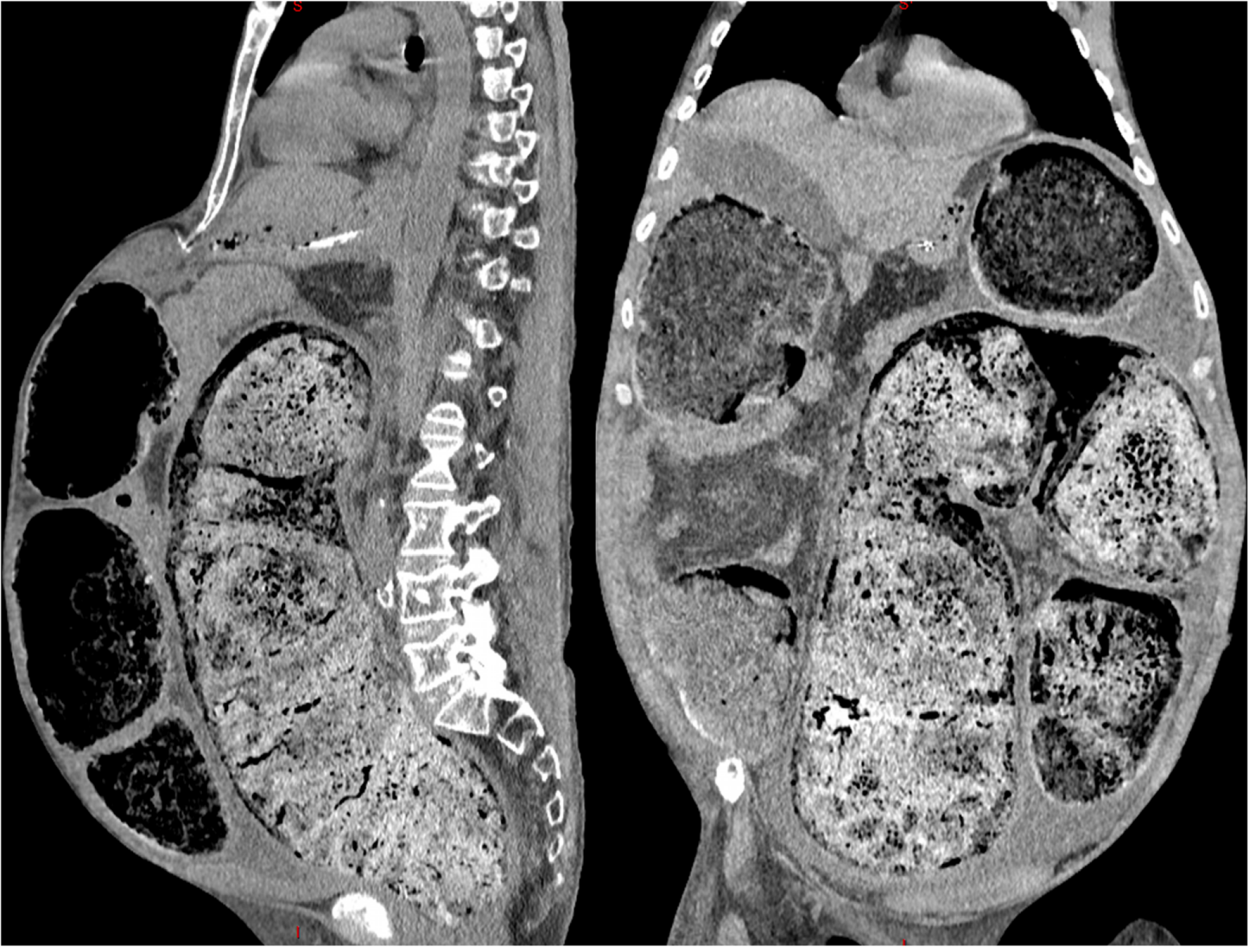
Saggital (left) and coronal (right) views of the abdominal computed tomography revealing a severe fecal impaction
